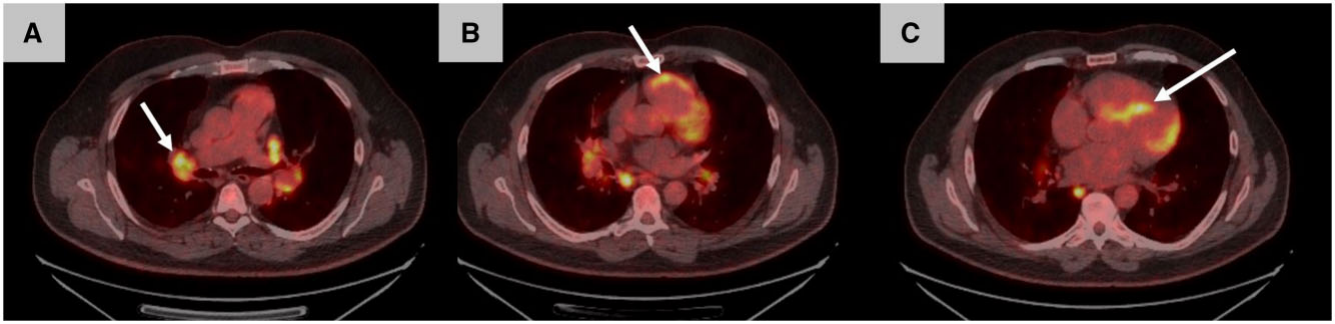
18-FDG-PET/CT showing cardiac sarcoid. 18-FDG-PET/CT transverse images showing multiple intensely avid areas of FDG uptake (A) in mediastinal and hilar lymph nodes, (B) in the right ventricle and (C) in the left ventricle, with the anteroseptum showing the highest level of FDG uptake. A diagnosis of cardiac sarcoidosis was made. 18-FDG-PET/CT: fluorine-18 fluorodeoxyglucose PET/CT; FDG: fluorodeoxyglucose.
